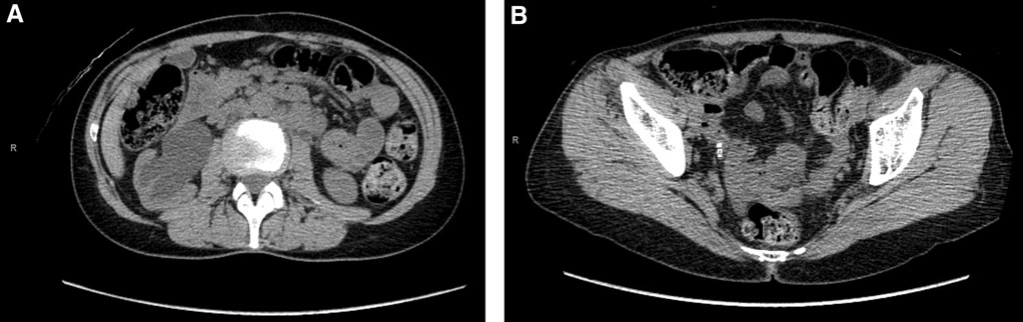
Noncontrast CT scan at initial presentation, demonstrating (A) severe hydroureteronephrosis and (B) a 2.5 mm distal ureteral stone.
